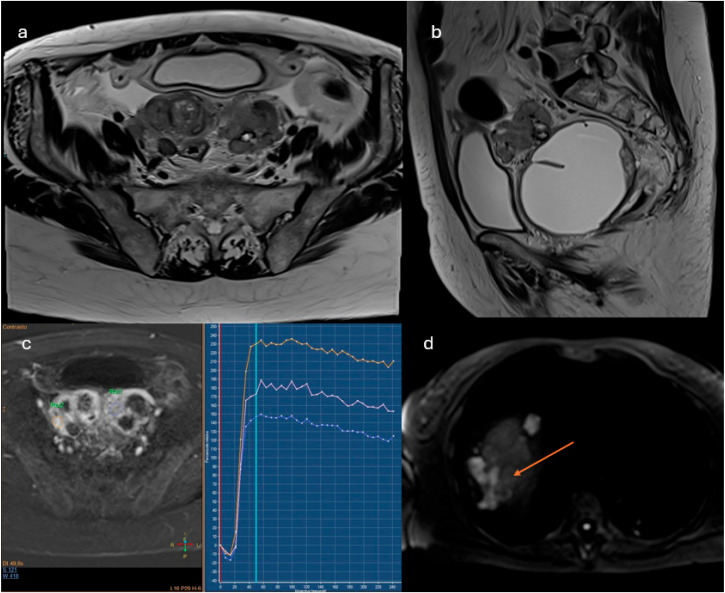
Bilateral adnexal masses (a,b). In DCE-perfusion ROI were placed in two different sites of the solid tissue ((c), orange and blue curves); the orange curve resulted “high risk curve” if compared to the curve of the myometrium ((c), pink curve): O-RADS 5. The study was extended at the upper abdomen with DWI, where peritoneal implants in subphrenic space were detected ((d), arrow).
